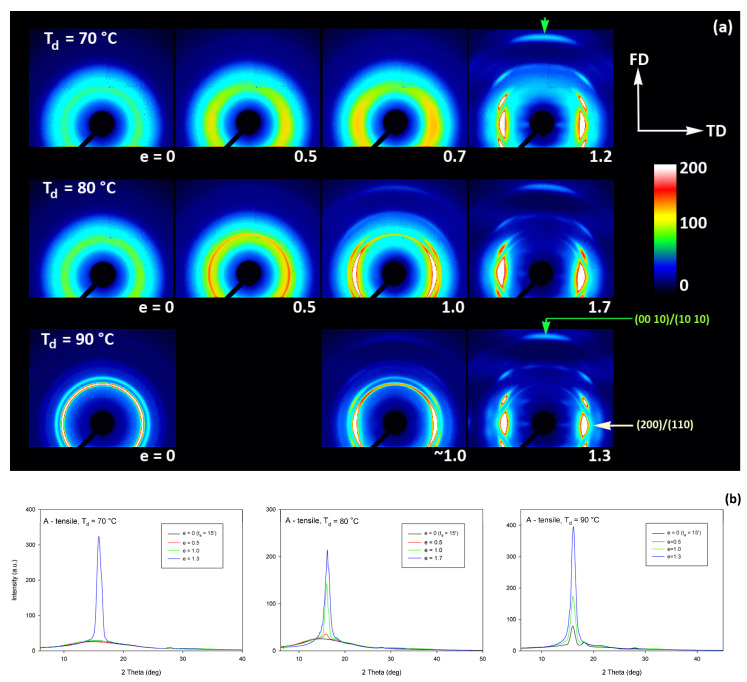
(a) 2D-WAXS patterns of the initially amorphous A samples deformed in uniaxial tension at temperature T_d_ = 70 °C (top row), T_d_ = 80 °C (middle row), T_d_ = 90 °C (bottom row) obtained in transmission with the X-ray beam illumination normal to the plane of the sample. The true strain indicated for each pattern is the local true strain calculated from the distance of the ink marks printed on the sample. (b) One-dimensional radial sections derived from 2D images along the transverse direction, TD—obtained by azimuth integration of 2D patterns in the azimuth range (−5°, +5°) around TD (ψ_TD_ = 0°).
